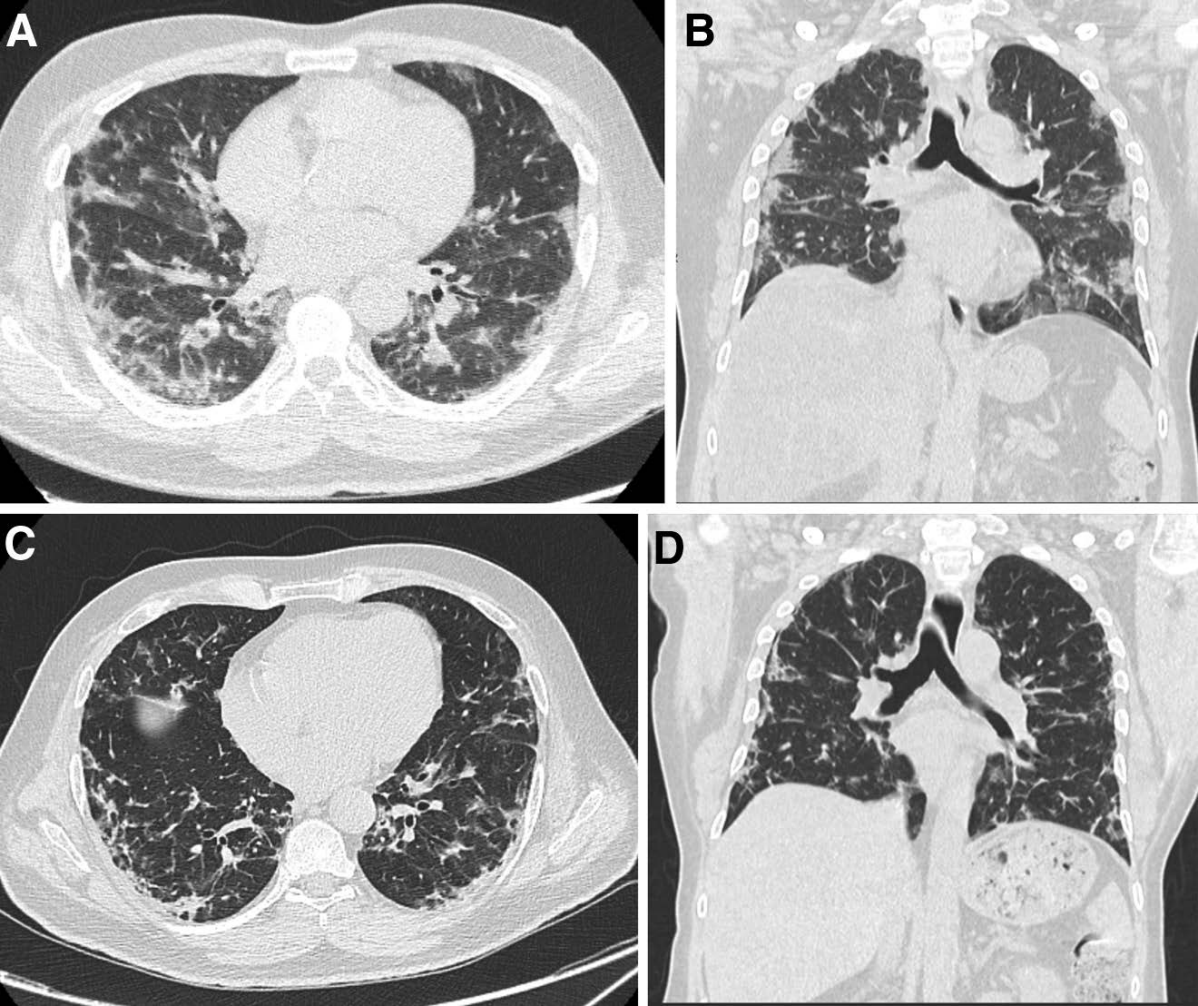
Patients confirmed positive for COVID 19, first day at admission CT chest showed. (A) Axial CT chest cuts showed bilateral multi-segmental sub-pleural patchy areas of consolidations with interstitial marking and fibrotic parenchymal bands (B) coronal reformate confirmed the bilateral multi-segmental sub-pleural patchy areas of consolidations with interstitial marking and fibrotic parenchymal bands (C and D) axial and coronal images in follow up after 16 d showed resolution of consolidation patches yet with residual interstitial marking, sub-pleural line and fibrotic parenchymal bands. CT = computed tomography.
